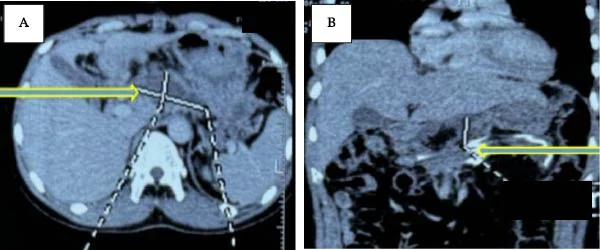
Preoperative abdominal CT scan findings in Case 2. (A) Axial arterial‐phase CT image demonstrating a well‐defined hypervascular lesion (arrow) measuring 11 × 11 mm at the pancreatic isthmus, with characteristic enhancement consistent with a neuroendocrine tumor. (B) Coronal reconstruction confirming the isthmic location of the lesion (arrow) in relation to the adjacent pancreatic parenchyma and peripancreatic vessels. All patient‐identifying information has been removed.
